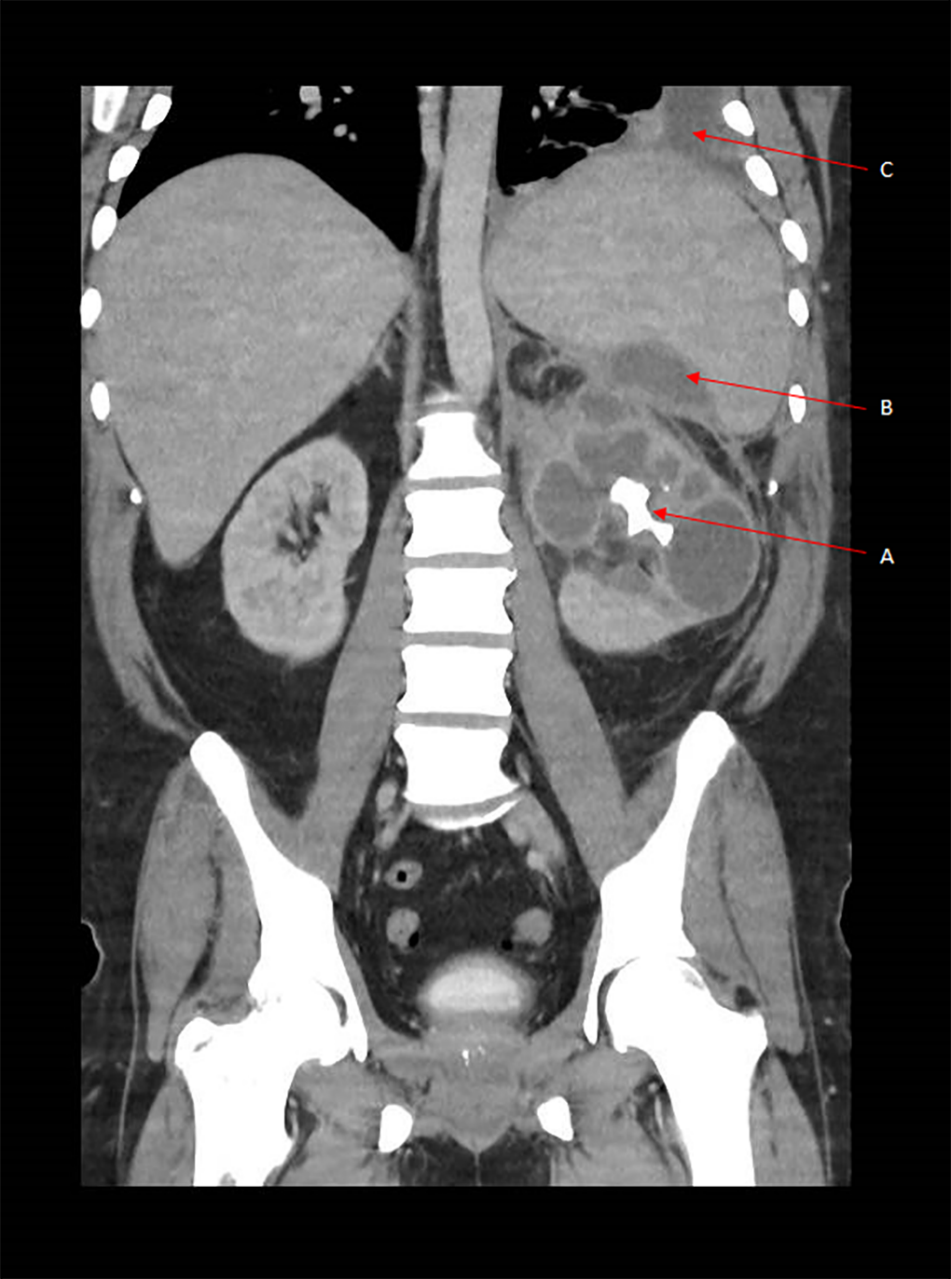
Coronal view of computed tomography imaging of the abdomen demonstrating xanthogranulomatous pyelonephritis, with a positive bear’s paw sign and a large obstructive renal staghorn calculus (A). A fluid‐filled collection is also seen at the hilum of the spleen (B). There is a moderate‐sized left pleural effusion with associated splenomegaly (C).
